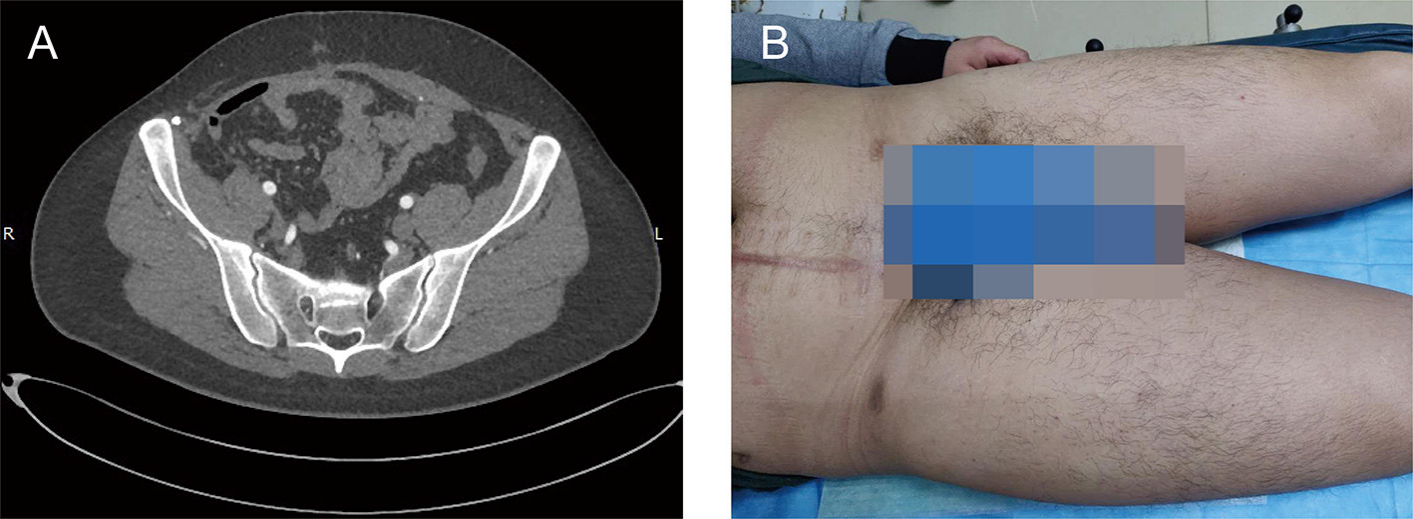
Clinical images at postoperative 20th month. (A) The postoperative contrast-enhanced CT showed no findings of recurrence. (B) Physical examination showed the swelling of right lower extremity.
